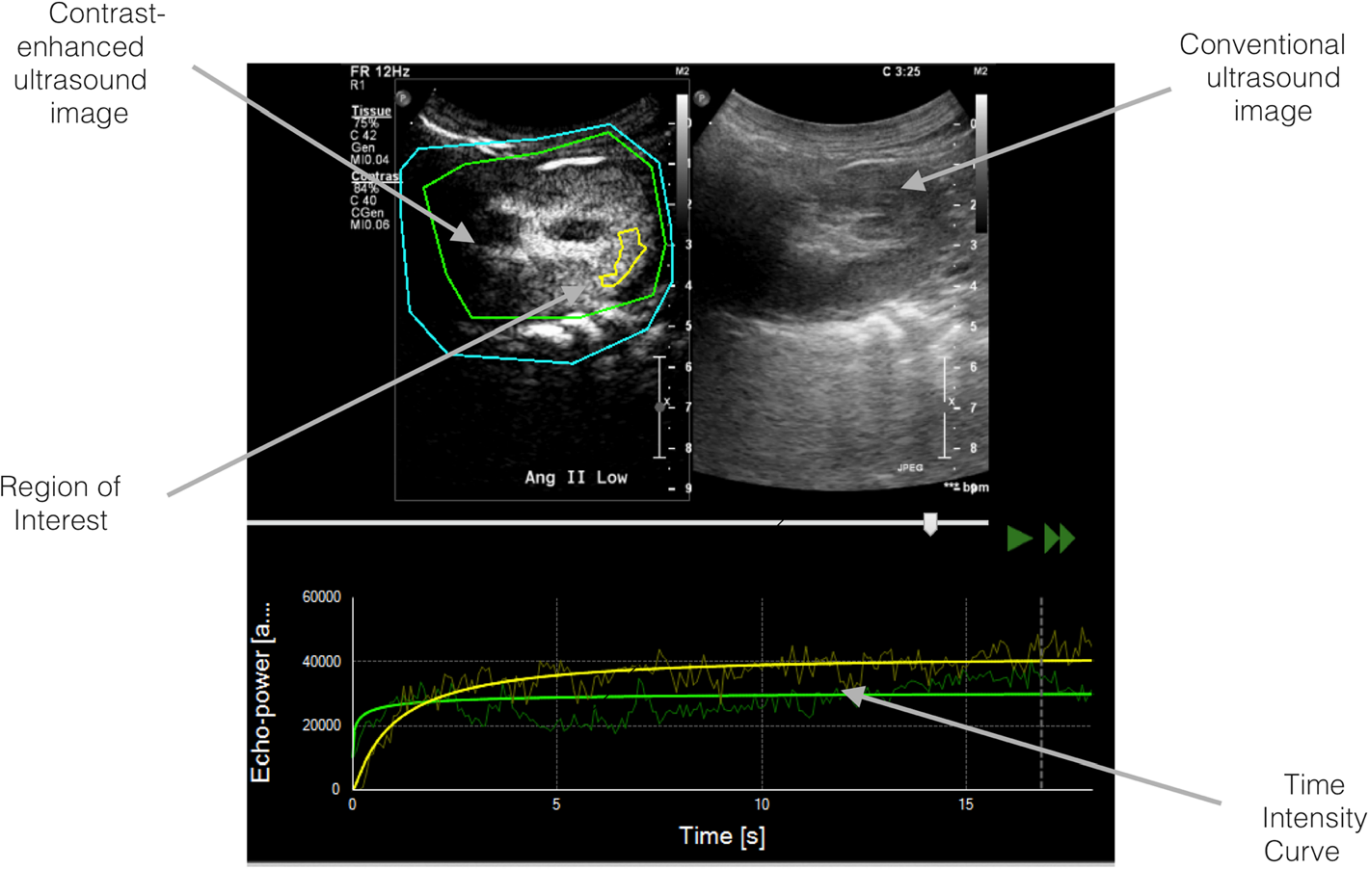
Software analysis data. Top left panel shows contrast-enhanced ultrasound images with region of interest drawn (yellow line). Top right panel shows conventional (B mode) imaging for anatomical localisation. Bottom panel shows linearized time intensity curve for the region of interest (yellow curve) and overall image (green curve_not relevant for perfusion quantification). AngII: angiotensine II.
